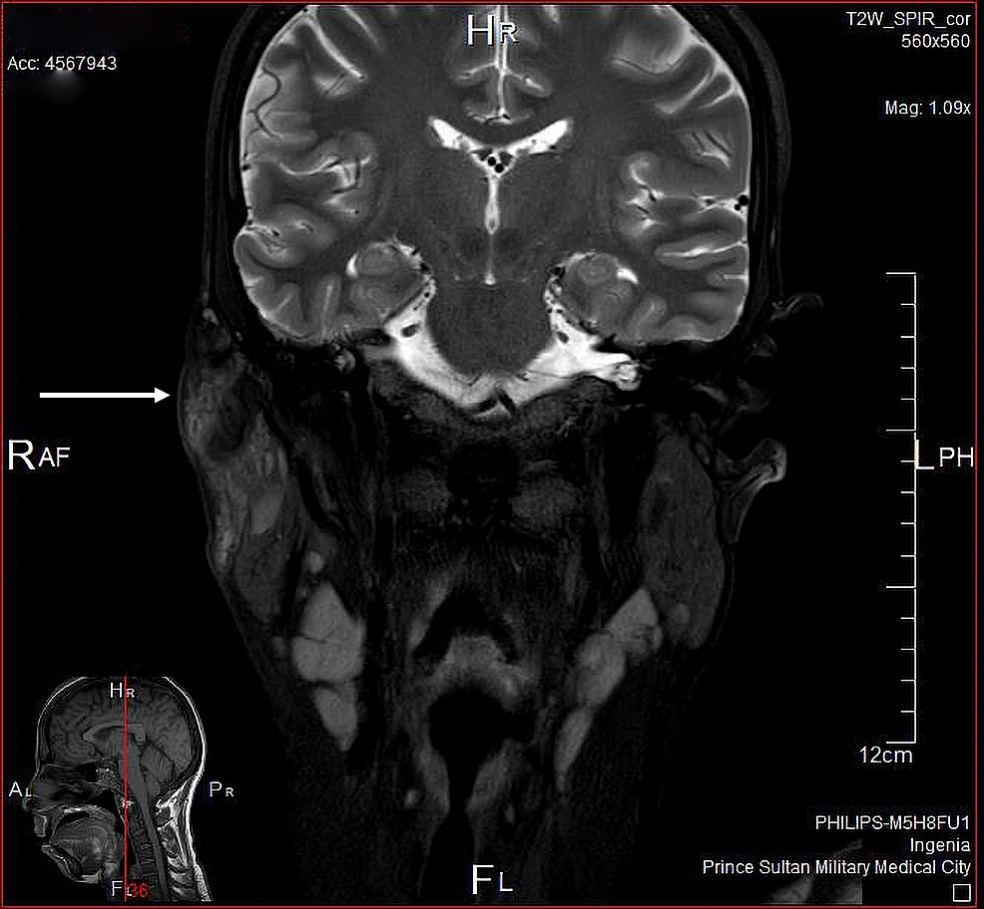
MRI showing infiltration of the overlying subcutaneous tissue - image 2 MRI: magnetic resonance imaging
